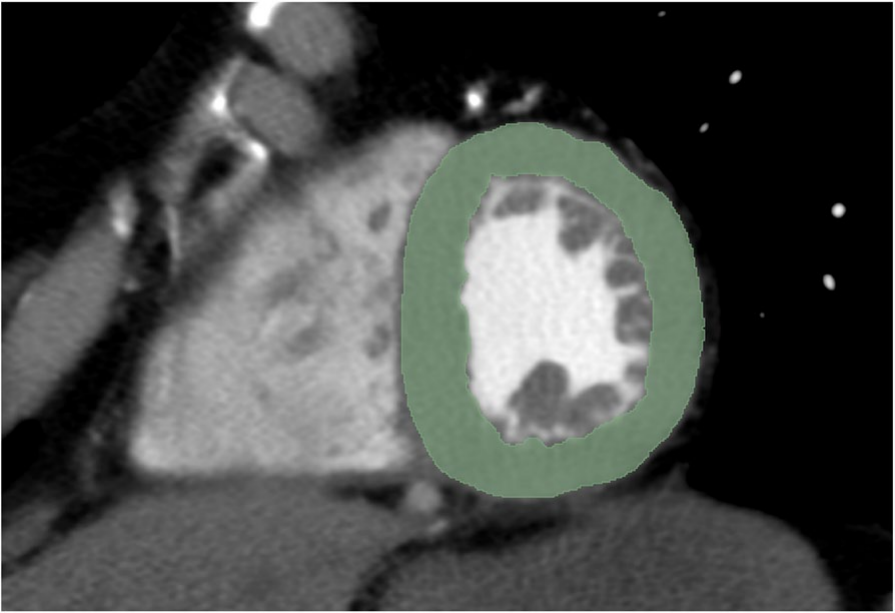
Representative example of myocardial segmentation in a patient PCD-CT scan at 70 keV
